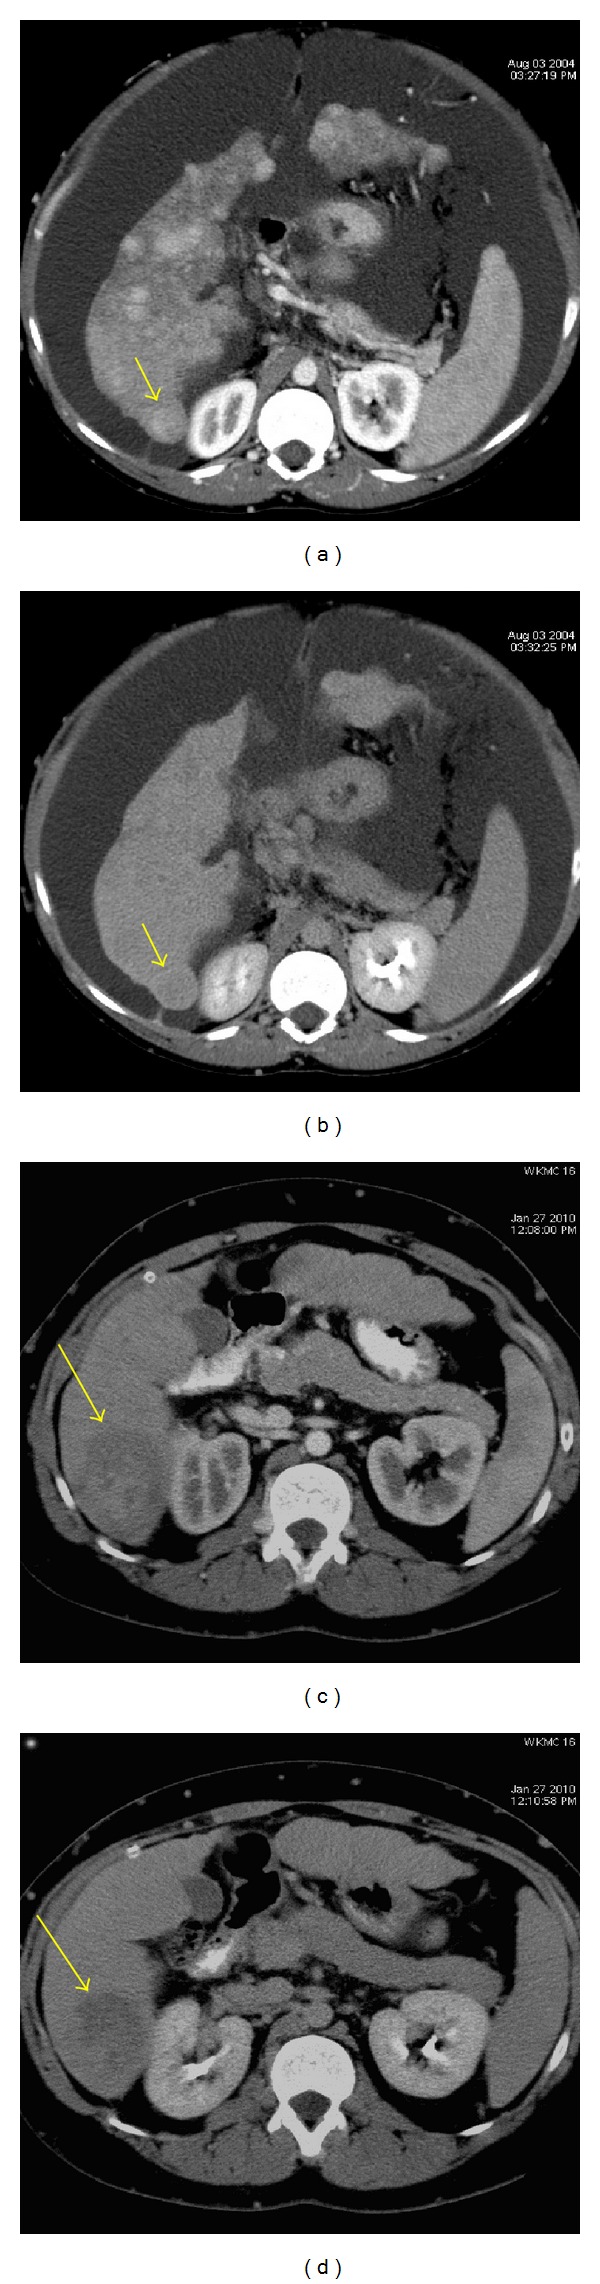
Dysplastic nodule in hepatic cirrhosis. Axial ((a), (b)) CT images during arterial and excretory phases show a dominant heterogeneous slightly hyperdense lesion in the segment VI compatible with dysplastic nodule (arrows); this lesion demonstrates a larger size compared with the remaining nodular hepatic lesions raising the possibility of DN in a patient with normal alpha feto protein value. Six-year imaging followup ((c), (d)) showing malignant transformation of this lesion (long arrows).
